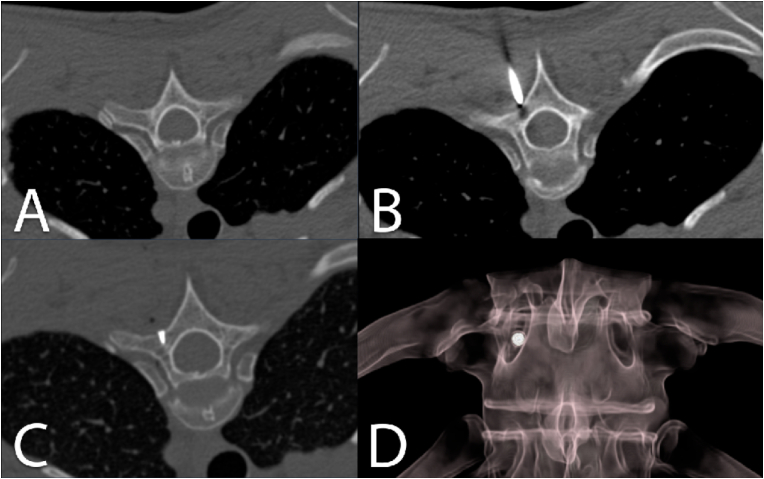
CT image documentation of screw marking in the thoracic spine. CT scan to locate the correct position (A). Positioning of the K-wire at the correct location (B). Documentation of the screw within the surgical trajectory (C). 3D-volume rendered reconstruction with the inserted screw in the index pedicle (D).
